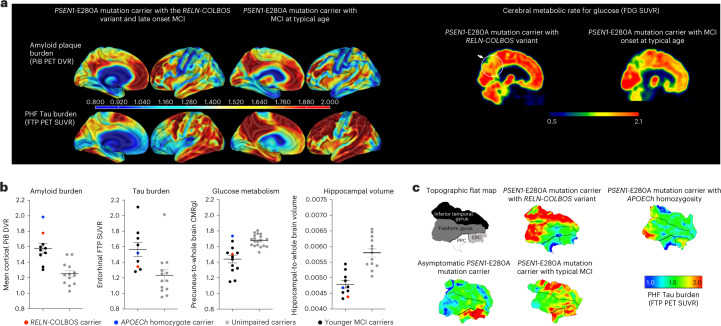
PET imaging of the *RELN-COLBOS* (H3447R) carrier. a, Representative PiB PET amyloid and FTP Tau PET imaging of the male case with *RELN-COLBOS* (left) compared to a *PSEN1-*E280A mutation carrier with MCI at a typical age (right). For both measurements, specific binding of the tracer is represented using a color-coded scale with blue being the lowest (DVR or SUVR = 0.8) and red being the highest (DVR or SUVR = 2.00) degree of binding. Right, representative FDG PET precuneus cerebral metabolic rate for glucose (CMRgI) of the male case with *RELN-COLBOS* (left) compared to a *PSEN1*-E280A carrier with MCI at a typical age (right). Binding affinity of the dye is represented using a color-coded scale with blue being the lowest (SUVR = 0.5) and red being the highest (SUVR = 2.1) degree of binding. PHF, paired helical filament. b, Dot plot analysis of the imaging measurements shown in a for amyloid and Tau burden, glucose metabolism and hippocampal volume. Brain imaging measurements of the male case with *RELN-COLBOS* (red dot) compared to the previously published *APOECh* homozygote female (blue dot), unimpaired *PSEN1*-E280A carriers (gray dots, *n* = 18 for the glucose metabolism panel, *n* = 13 for all other panels) and younger carriers of the MCI *PSEN1*-E280A mutation (black dots, *n* = 7 for the Tau burden plot, *n* = 8 for the amyloid burden and hippocampal volume plots, *n* = 11 for glucose metabolism)^2^. Some previously published data points are included in the figures because they are the only available data for comparison^2^. Data are expressed as individual values with the mean ± s.e.m. c, Anatomical details of Tau burden in the temporal cortex. Flat map representations of the right hemisphere temporal lobe cortex for regions of interest (ROIs) (top left, ERC), with Tau PET (FTP) overlay for four cases. The asymptomatic *PSEN1*-E280A carrier was 38 years old; the *PSEN1*-E280A carrier with typical MCI was 44 years old. The male carrier of *RELN-COLBOS* was notable for having relatively lower Tau burden in the medial temporal regions (ERC and PPC), compared to typical *PSEN1*-E280A mutation carriers.
